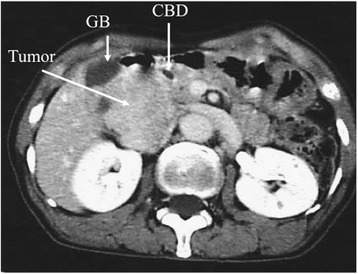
Abdominal computed tomography. Contrast-enhanced abdominal computed tomography demonstrated a large heterogeneous mass, 5 cm in length that was compressing the IVC and head of the pancreas. *GB* gall bladder, *CBD* common bile duct, *IVC* inferior vena cava
